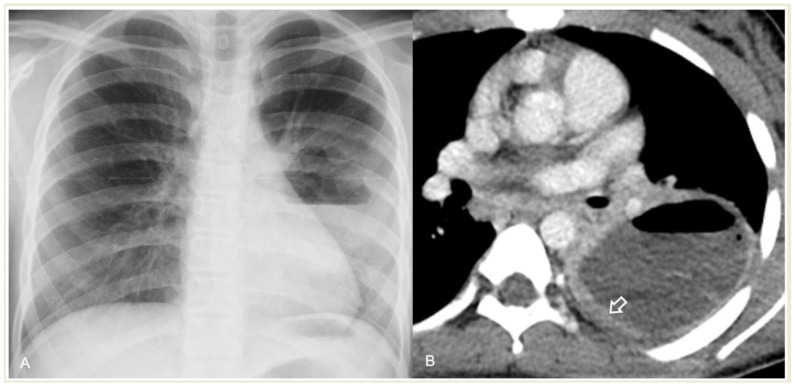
Lung abscess complicated CAP in a 12-year-old girl: (A) chest radiograph shows a cavity with an air–fluid level; (B) contrast-enhanced CT scan demonstrates a cavity with a thick enhancing wall (arrow) containing an air–fluid level, favoring the diagnosis of an abscess.
